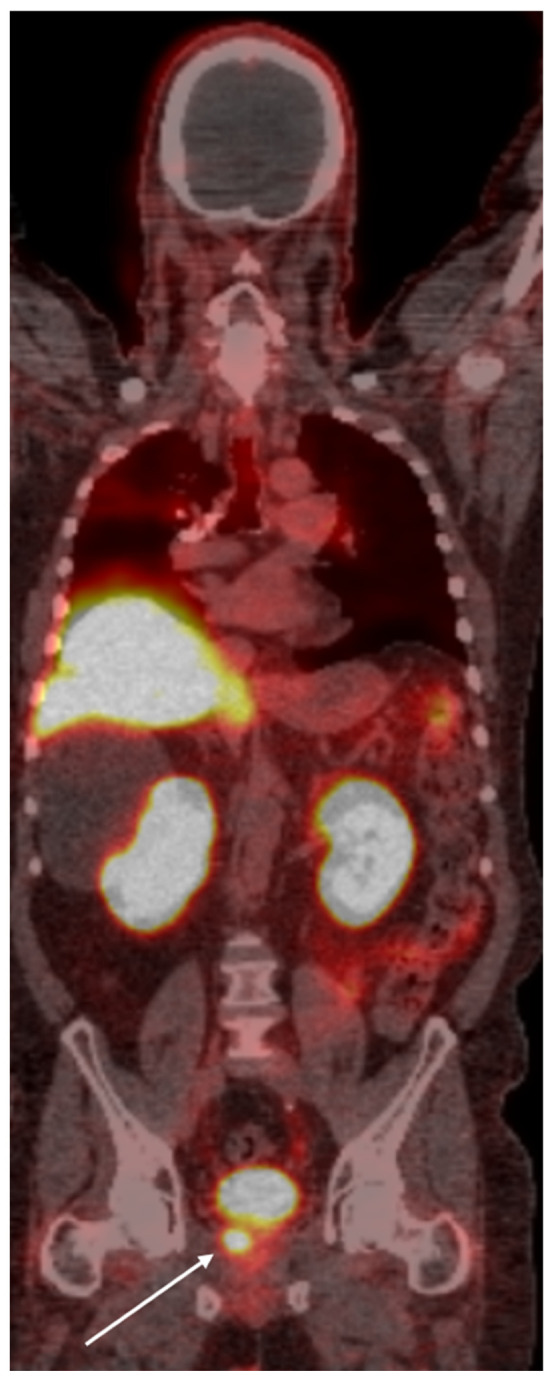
PSMA PET scan of a 66-year old demonstrates radiotracer uptake lesion in the right prostate bed (arrow), consistent with primary prostate carcinoma.
